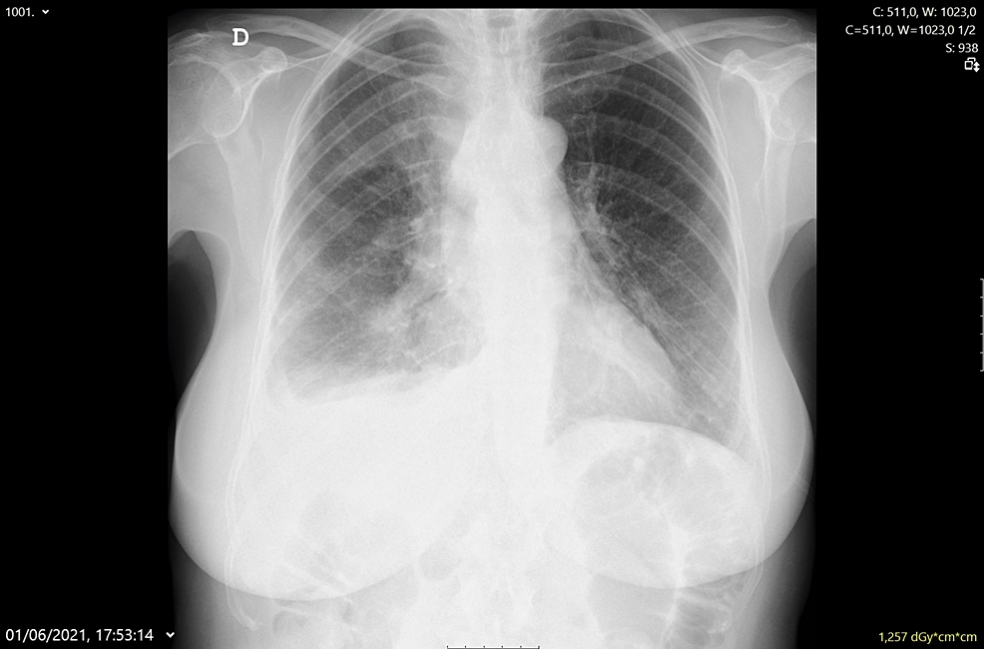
Thoracic X-ray after thoracocentesis
